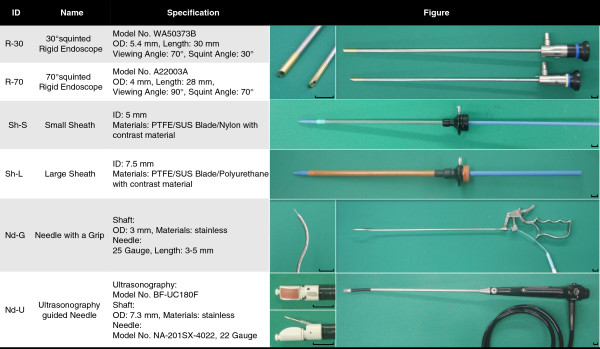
Characteristics of the devices for pericardial endoscopic surgery. We developed unique tools for pericardial endoscopic maneuvers. The ID, name, specification, and figure of devices are shown. R-30 and R-70 were originally designed for transurethral cancer resection with a 30 and 70° squinted visual field. Sh-L and Sh-S are 15 French and 22.5 French sized sheaths, respectively, with guide wires clamps. Nd-G is the cell transplantation needle with a grip and Nd-U is visible under ultrasonography. Black scale: 1 cm; ID: inner diameter; OD: outer diameter; PTFE: polytetrafluoroethylene.
